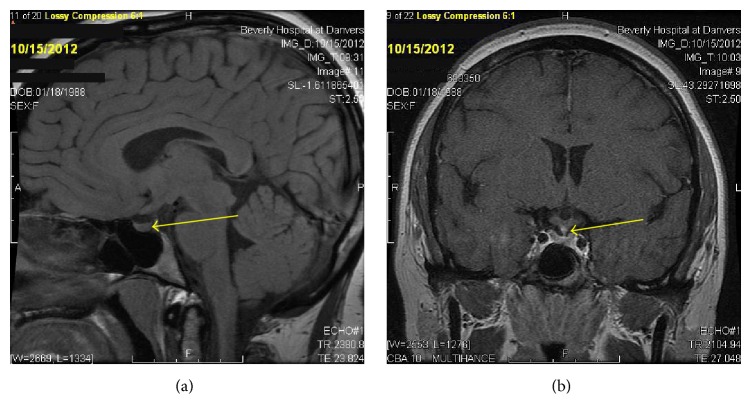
MRI shows absence of posterior pituitary bright spot (a) and thickened pituitary stalk (b).
